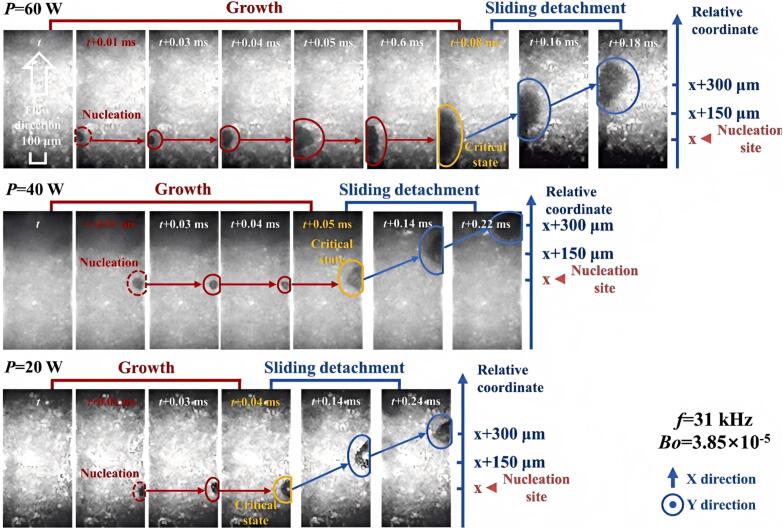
Bubble behavior under different ultrasonic powers [102].
